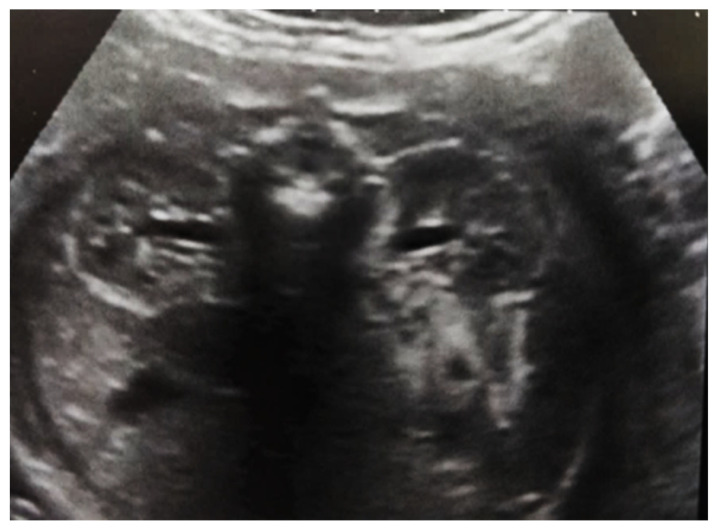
Ultrasound at 32 weeks of gestation. Bilateral pyelectasis.
